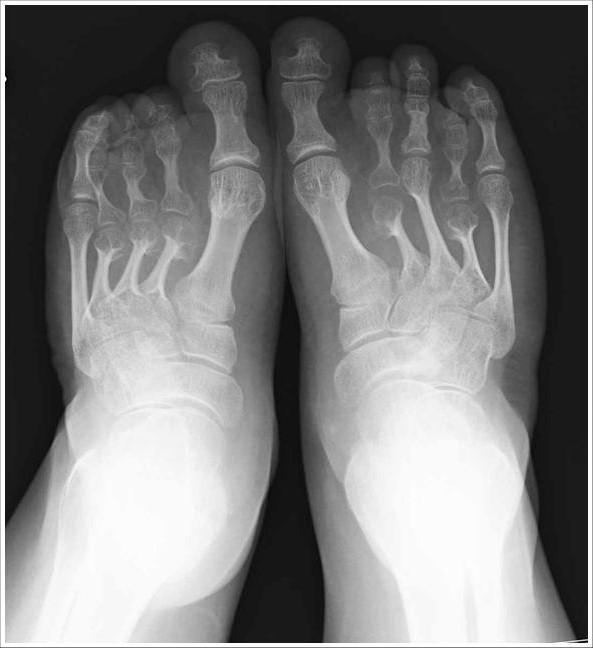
Radiographs of feet. Note the 2^nd ^and 4^th ^metatarsal bones and the great toe.
